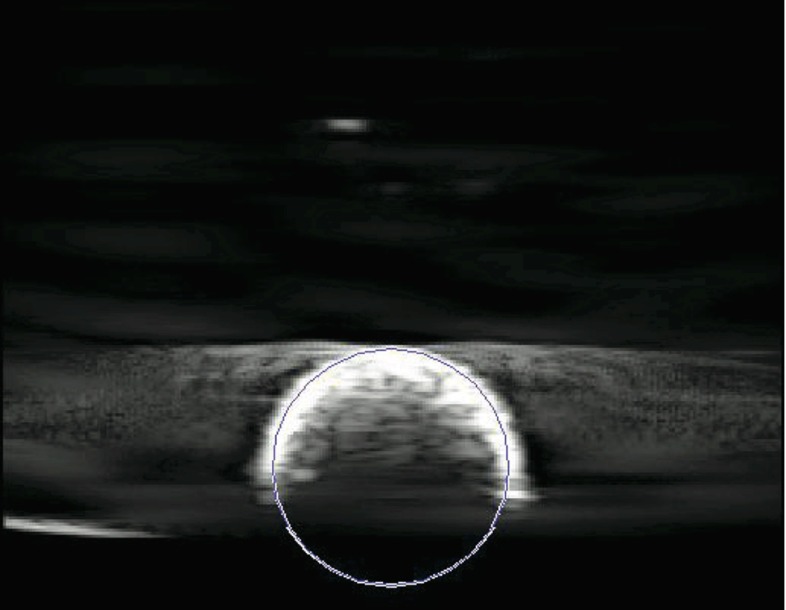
An ultrasound image cutting through the imaging target (plastic sphere) during probe calibration. The location of the sphere center within that image was found by adjusting the position of a virtual circle of the same diameter as the sphere, until its circumference corresponded with the surface of the imaged sphere
